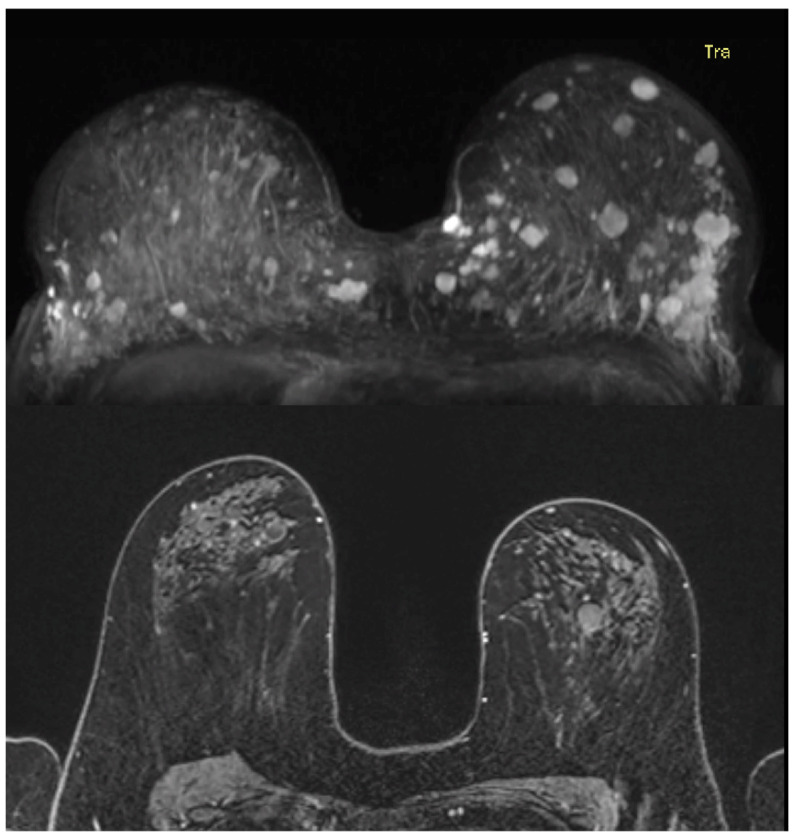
MRI examinations of two women with multiple benign breast lesions in both breasts.
